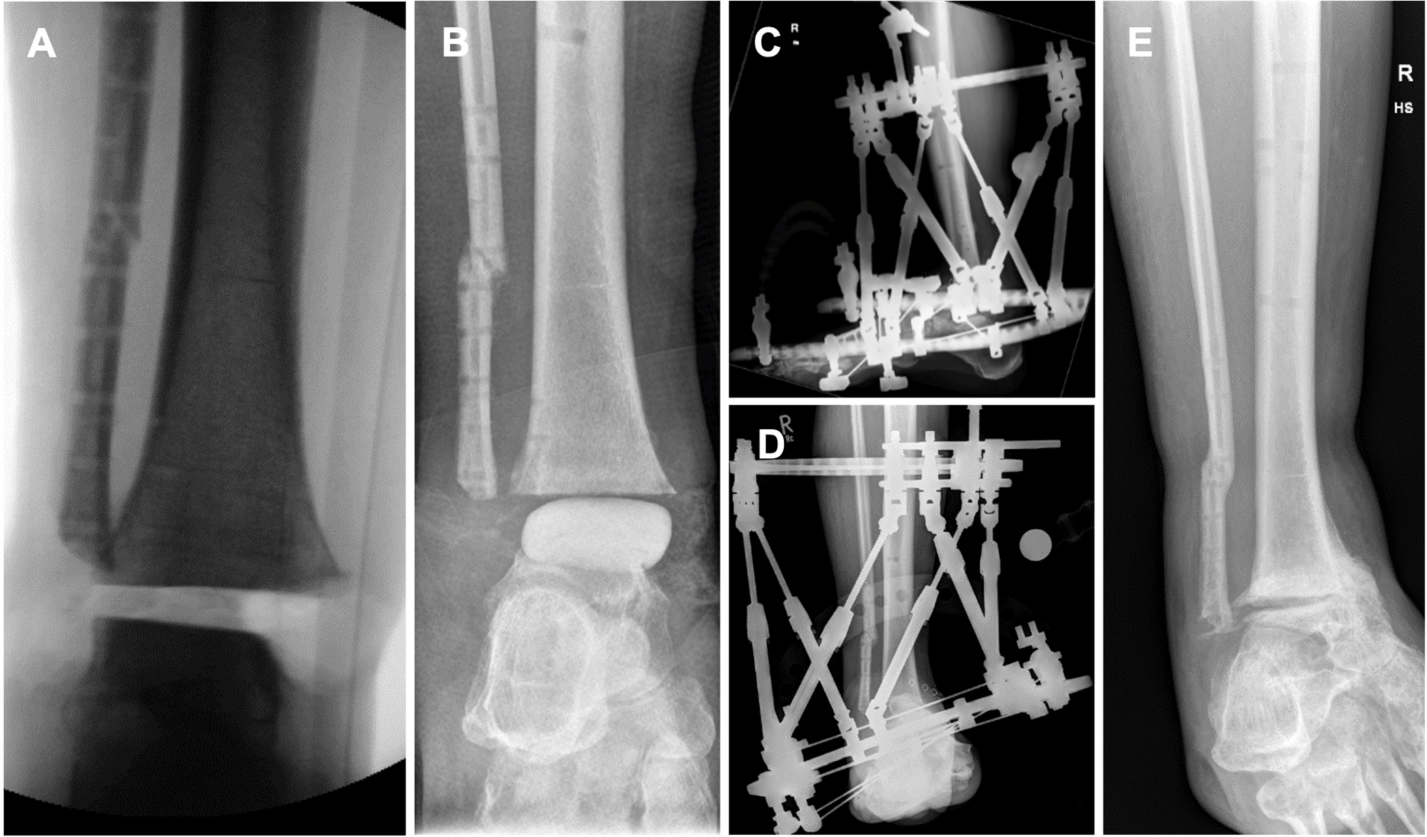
Radiographs of the lower leg (A) Resection of the upper ankle joint, including the medial and lateral malleolus, and removal of all foreign implants. (B) Amphotericin B and vancomycin-infused PMMA spacer during the debridement phase. (C, D) Hexapod fixator to correct varus deformity. (E) Partial bone union after removal of the hexapod fixator.
